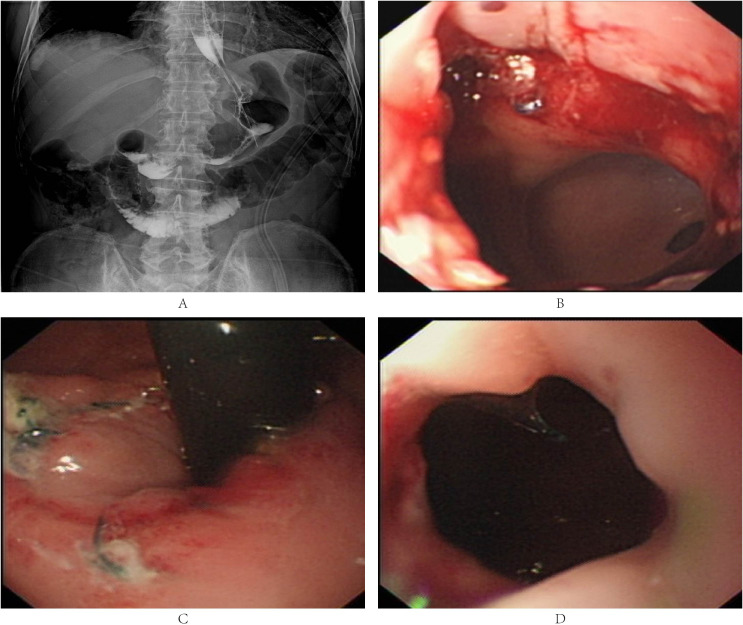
(A) Contrast radiography after LPG-DFT, revealing no anastomotic stenosis or anastomotic leakage. (B-D). Endoscopic findings after LPG-DFT, showing no patients were suffered from reflux esophagitis (Los Angeles Grade B or more) or anastomotic stenosis.
